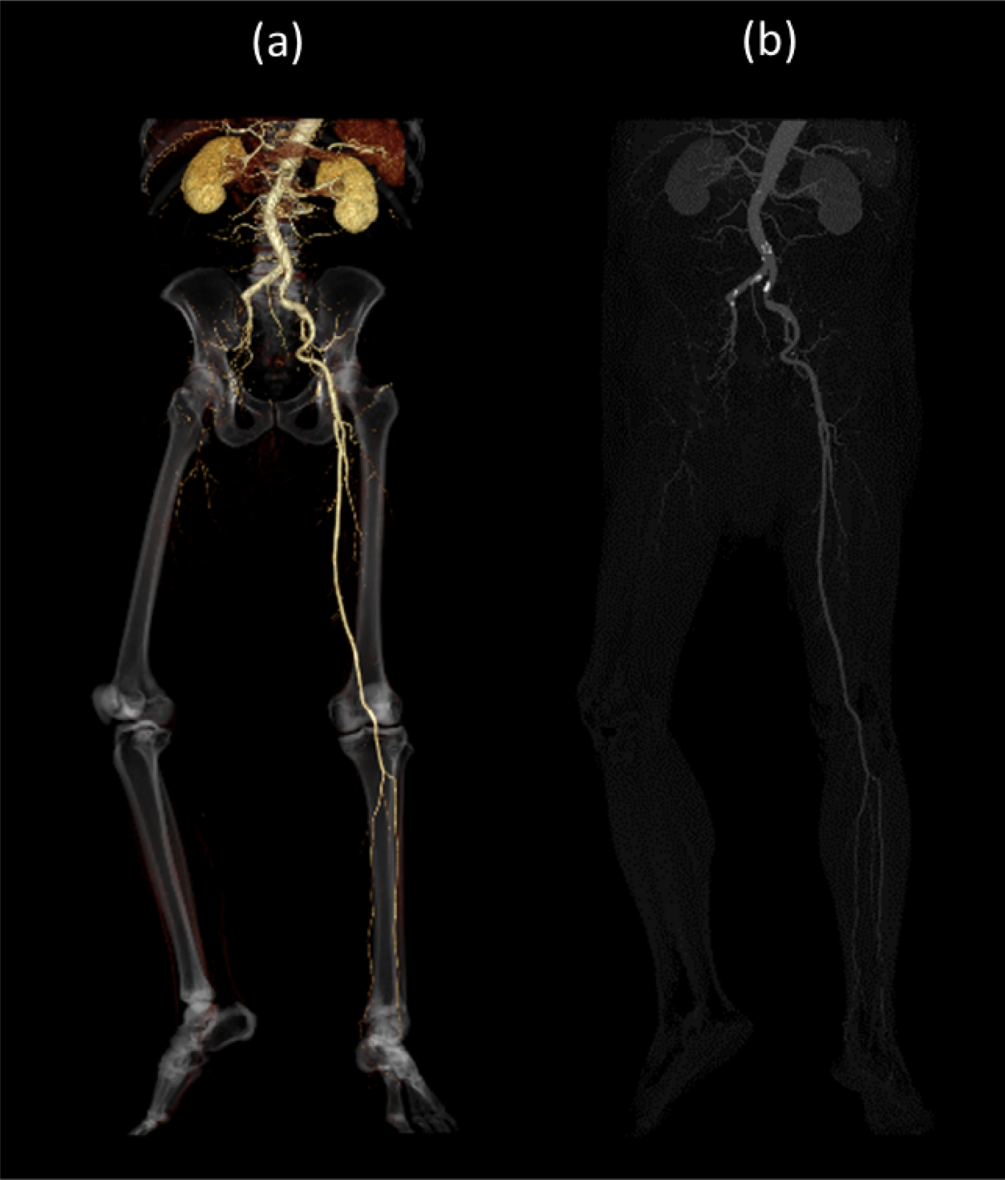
Three-dimensional CT angiography (a, b) showing an arterial occlusion extending from the common iliac artery to the right external iliac artery.
